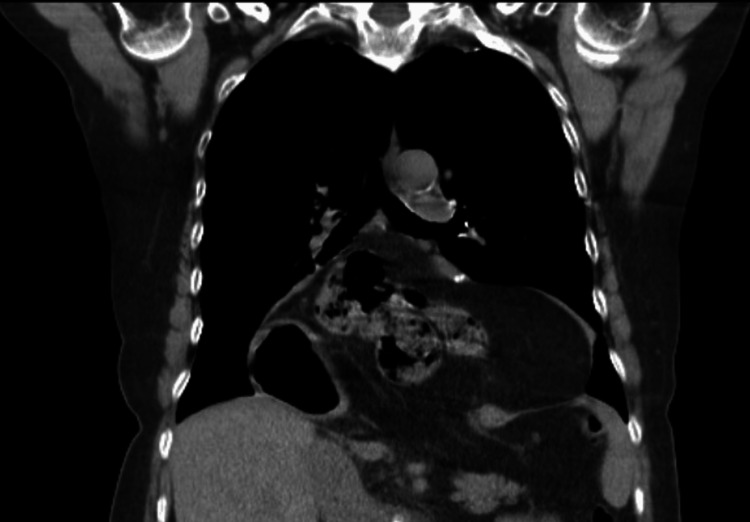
Type IV hiatal hernia demonstrated by CT CT: computed tomography
